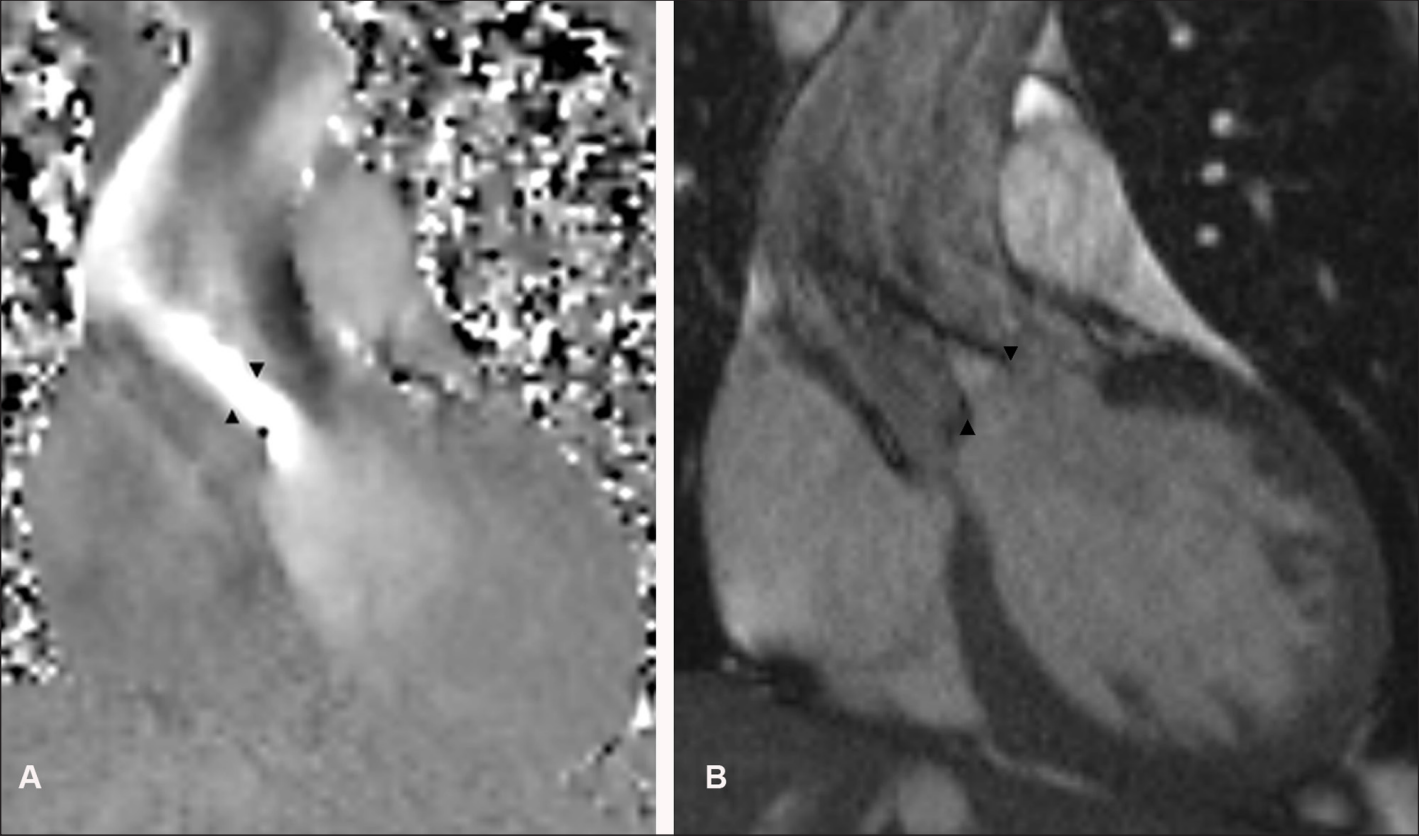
(A) Coronal flow velocity encoded images show high velocity blood flow across the aortic valve (arrowheads) compatible with a moderate aortic valve stenosis; a peak systolic velocity of 328 cm/s was measured. (B) Coronal cine MRI images of the left ventricular outlet during systole demonstrate a narrowed aortic valve orifice (arrowheads) during systole because of concurrent aortic stenosis.
